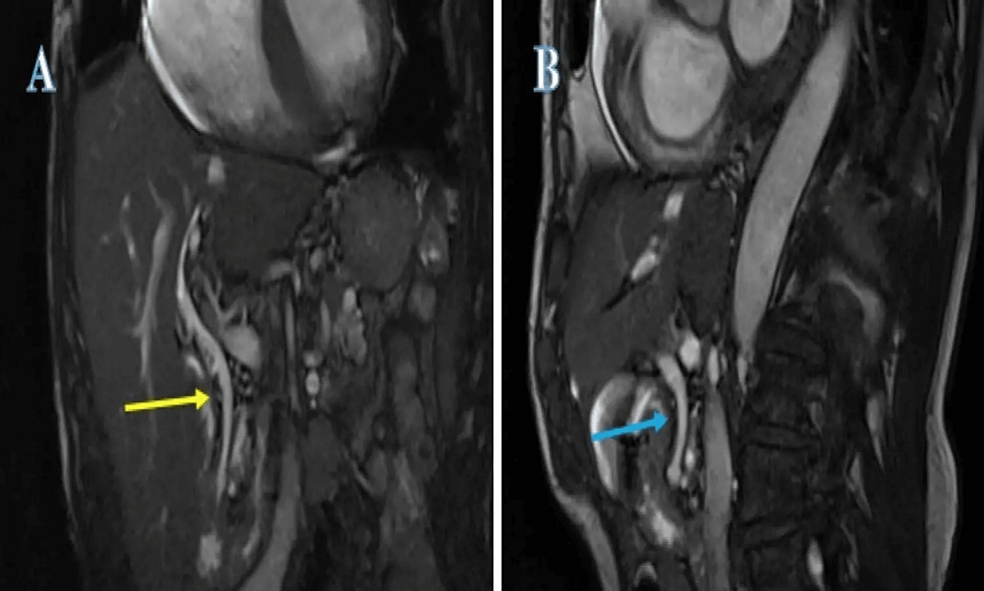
Repeat MRCP. (A) Coronal T2-weighted true fast imaging with steady-state free precession (TRUFI) image with fat saturation demonstrating normal calibre CBD free of stones (yellow arrow); (B) Coronal T2-weighted TRUFI image with fat saturation demonstrating normal calibre CBD free of stones (blue arrow)
